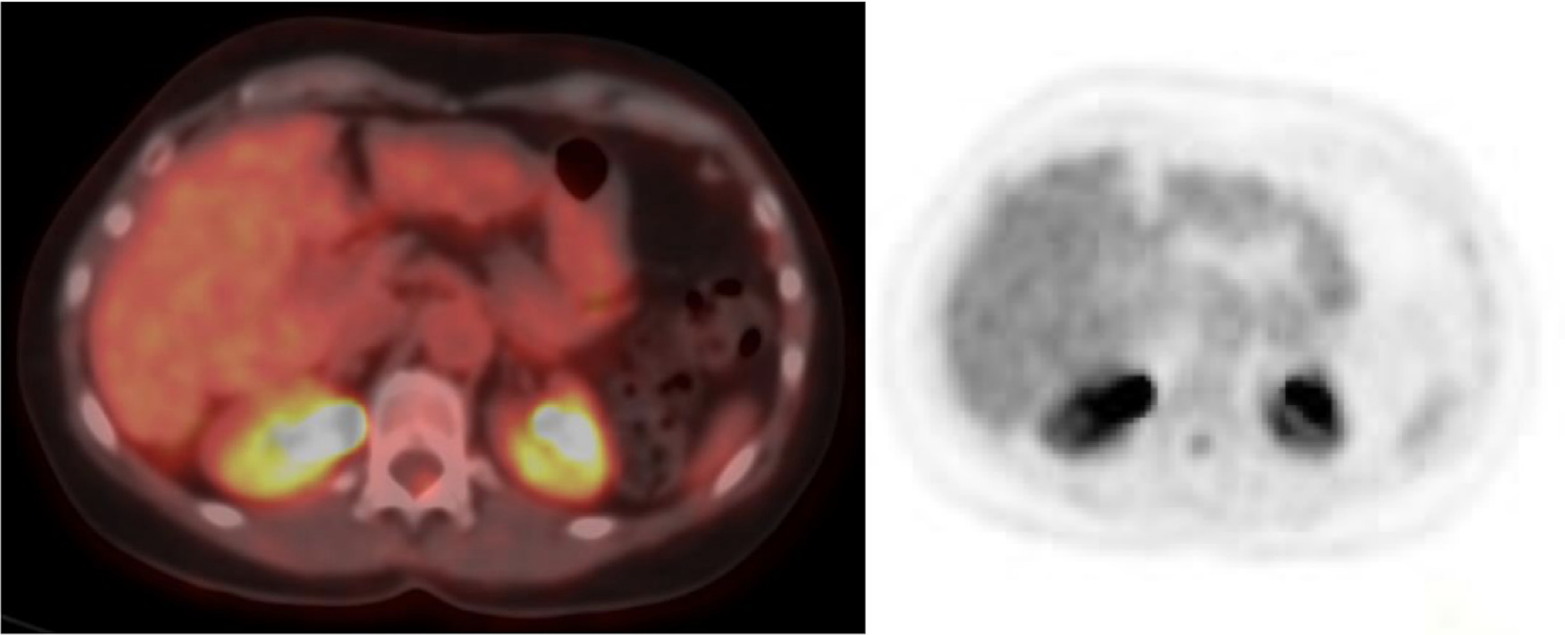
Positron emission tomography/computed tomography (PET-CT) with (18)F-fluorodeoxyglucose (18F-FDG) did not demonstrate pathological uptake of the radiopharmaceutical in the liver at the sites described in the above examination.
